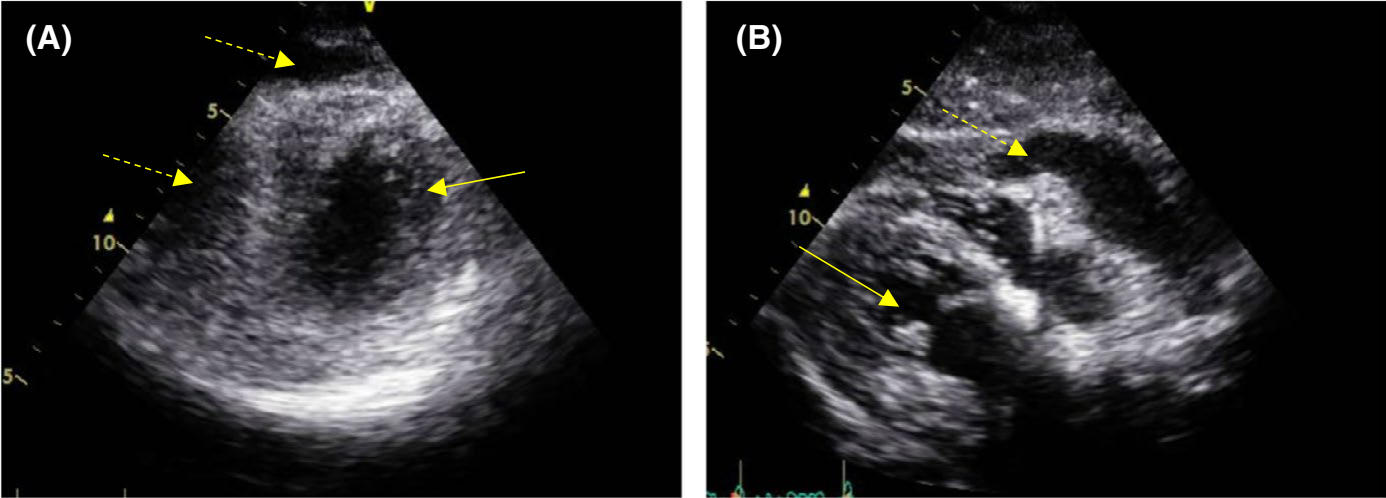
Echocardiography on admission. A, Image with the left ventricular short axis showing impaired RV filling due to cardiac tamponade. B, Image of the long axis of the left ventricle showing a large amount of pericardial effusion. ←, shows left ventricular; ⇠,shows pericardial effusion
